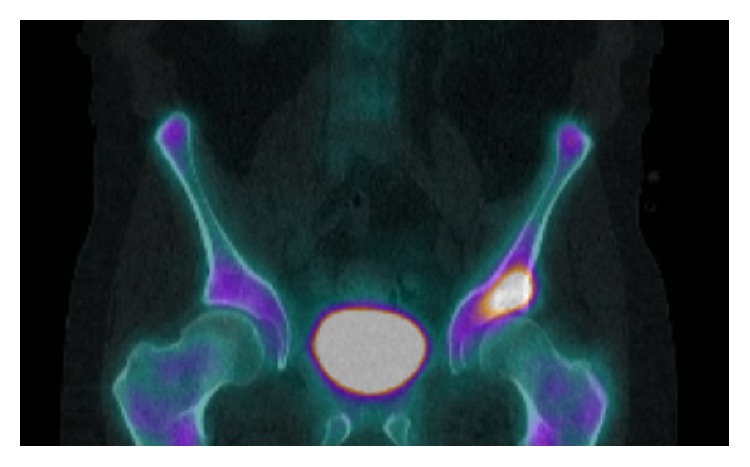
Delayed-phase SPECT/CT displaying increased activity in the left AIIS extending to the superior acetabular rim.
